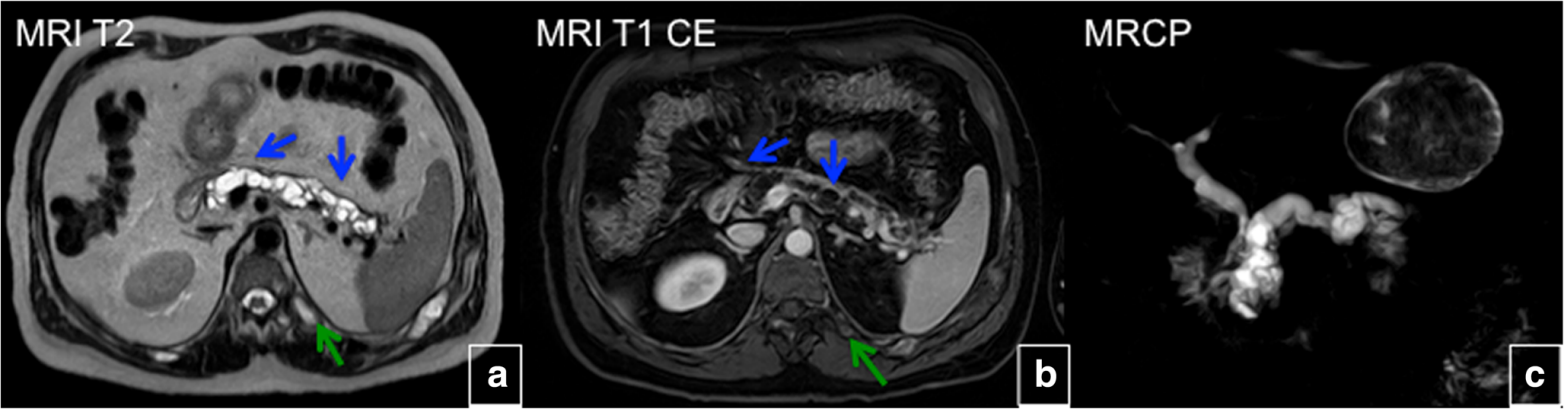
Intraductal papillary mucinous neoplasms (IPMNs) of the pancreas in McCune-Albright syndrome (*blue arrows*). T2-weighted MRI (a), T1 contrast-enhanced MRI (b). Coronal maximum intensity projection from a 3D T2-weighted MRCP acquisition shows IPMNs (c). Please note, a fibrous dysplasia lesion in the left rib (*green arrows*)
